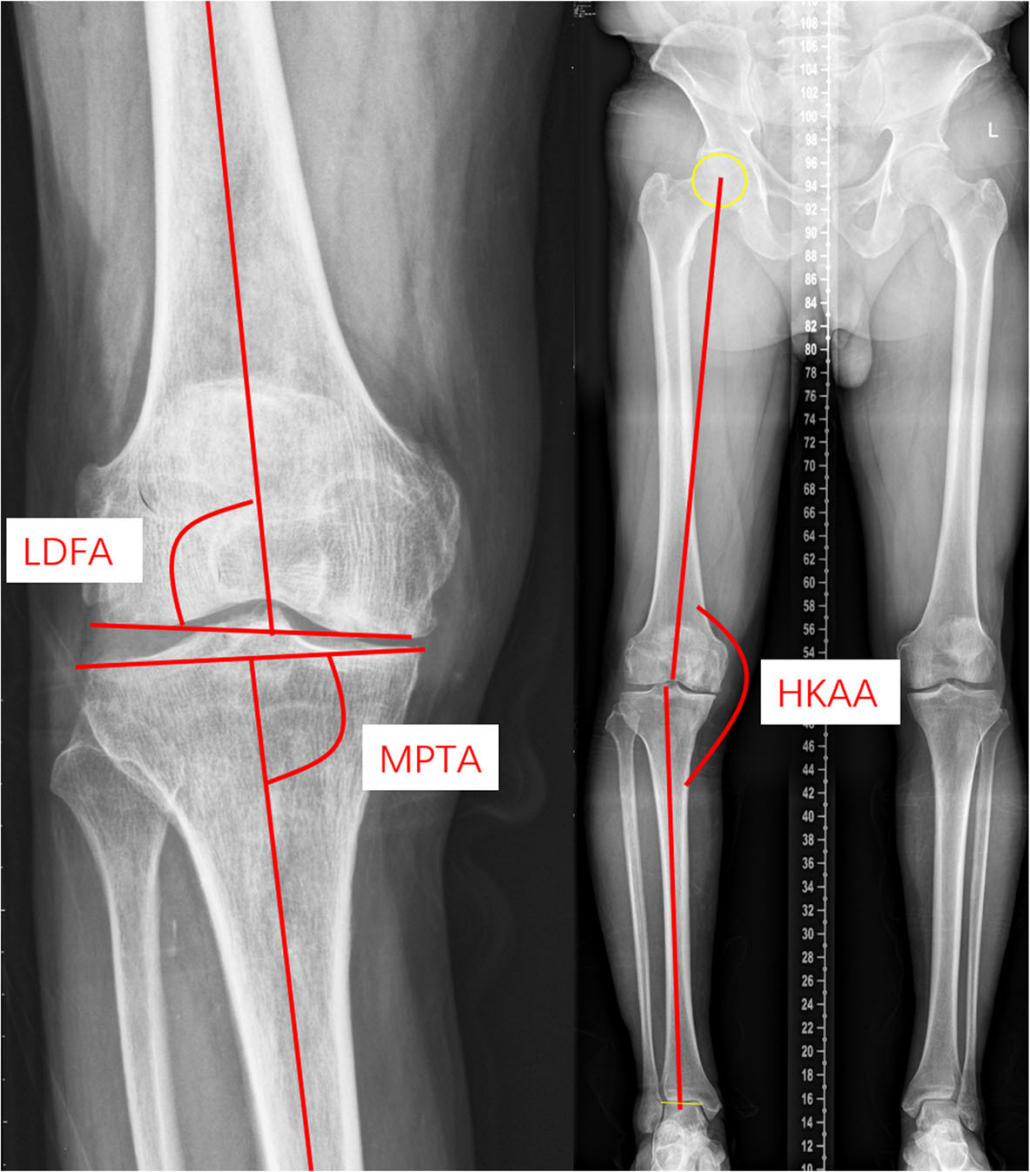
The patients were radiographically assessed using their preoperative weight-bearing X-rays. The overall limb alignment hip-knee-ankle angle (HKAA) was defined as the angle created by the hip center, center of the distal femoral notch, and center of the ankle talus. The lateral distal femoral angle (LDFA) was measured as the lateral angle between the distal femoral articular surface and the anatomical axis of the femur, while the medial proximal tibia angle (MPTA) was defined as the medial angle between the knee joint line of the tibia and axis line of the tibia
